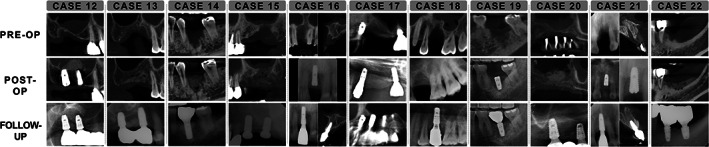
Radiographic findings for cases 12–22, including their preoperative, postoperative, and latest follow‐up data
